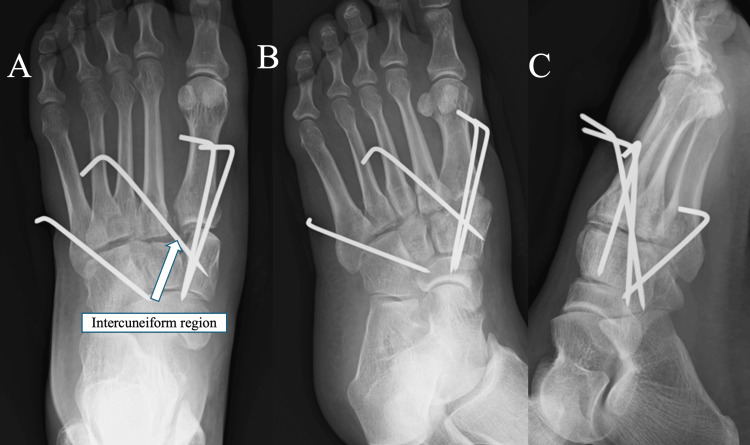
Preoperative radiographs of the left foot after initial emergency surgery (A) Anteroposterior view. (B) Medial oblique view. (C) Lateral view. The images show temporary percutaneous fixation after attempted closed reduction. Arrows and markers indicate the alignment of the medial column and the intercuneiform region, which should be carefully assessed when reduction is incomplete or difficult to maintain.
